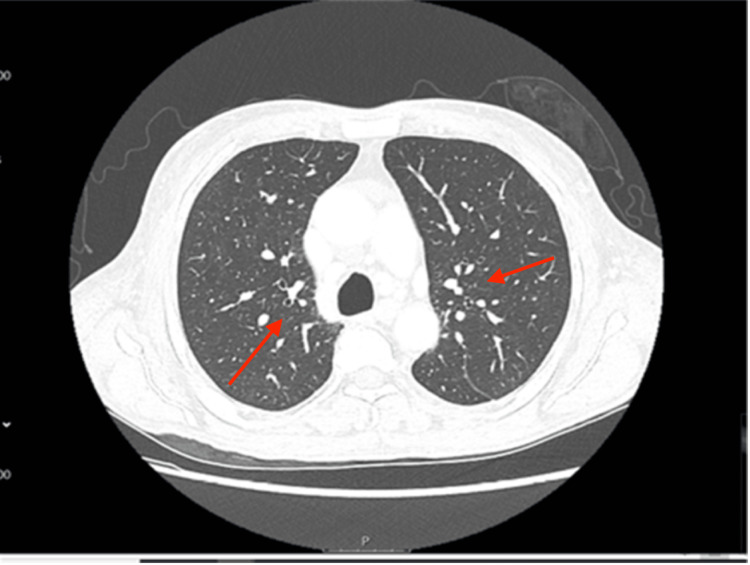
CT thorax with contrast showing interstitial pneumonia pattern of interstitial lung disease (red arrow). The image revealed no pulmonary nodules, masses, or focal airspace consolidations. However, the underlying pulmonary infectious process could not be excluded. In addition, the image revealed cardiomegaly with pericardial effusion and mediastinal lymphadenopathy. CT: computed tomography.
